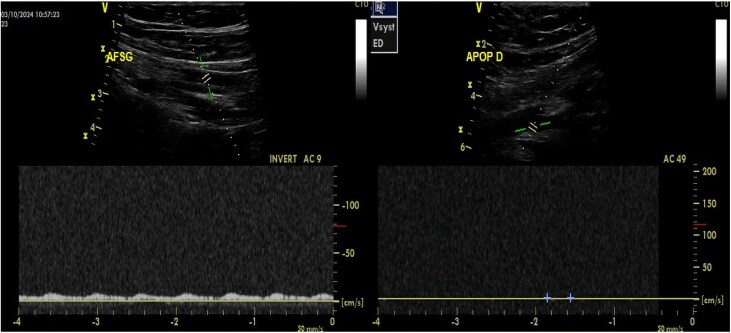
Arterial echo-Doppler images of both lower limbs showing pathological flows.
